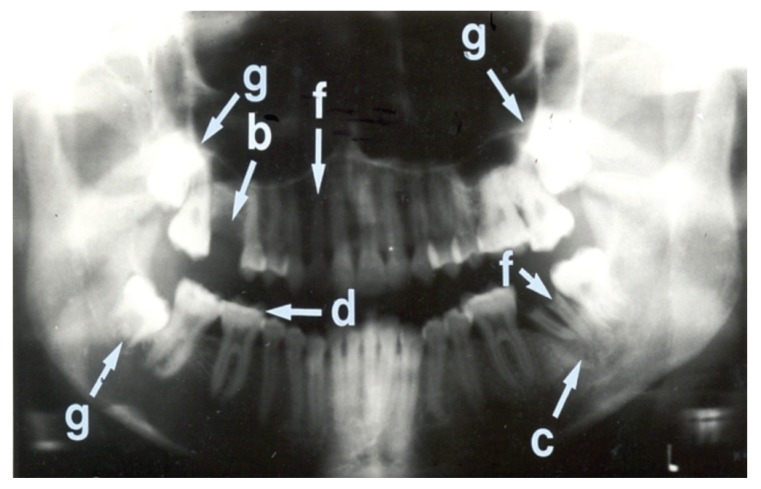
Panoramic radiograph showing more abnormalities than Fig. 1. This radiograph shows three impacted third molar teeth (g). The right first maxillary molar tooth is missing (b). The crown of the left second mandibular molar tooth is missing and retained roots are present (f). The same tooth also shows a peri-apical radiolucency (c) with a well-defined calcified margin indicating possible peri-apical cyst formation.^21^ The retained root of the right second maxillary incisor tooth is also seen (f). The occlusal surfaces of the right mandibular first and second molar teeth show attrition (d).
